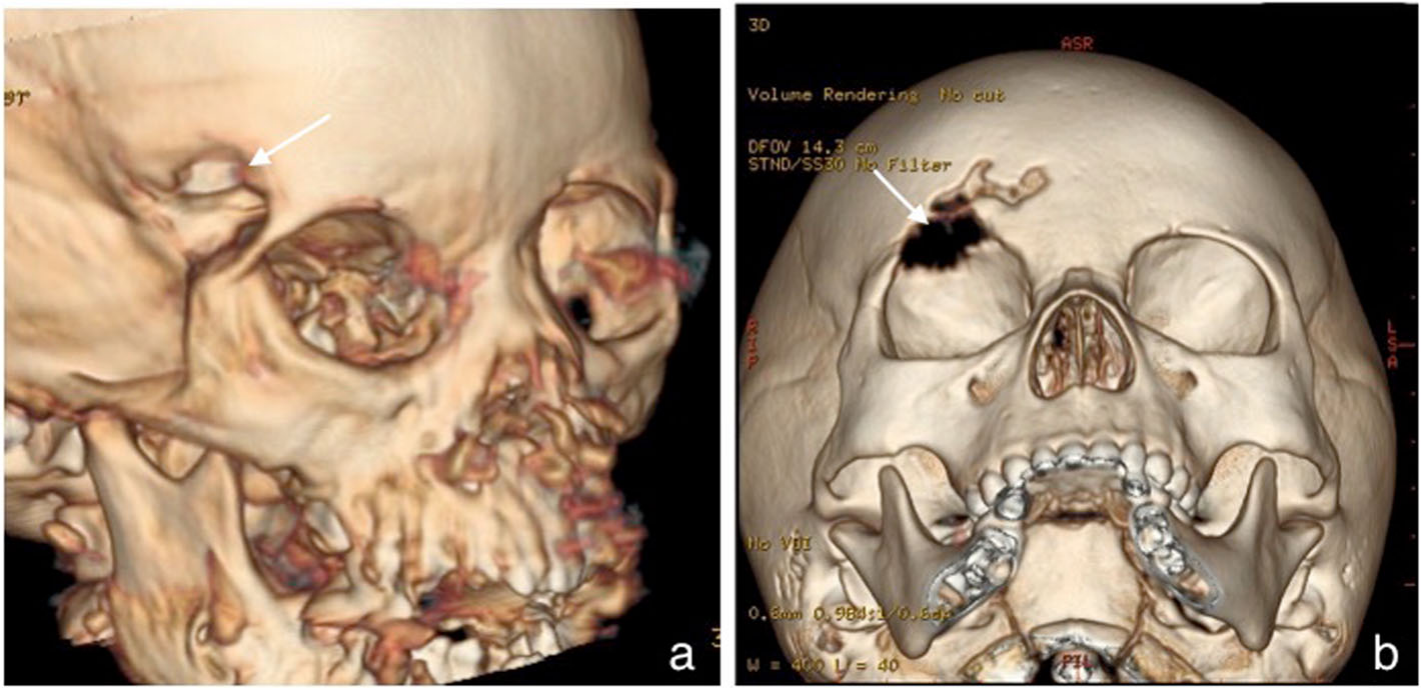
Representative 3D reconstructions on CT data: it can show the lesion site very well. a: lesion located the superlateral roof of the right orbit, b: lesion located the superior roof of the right orbit
